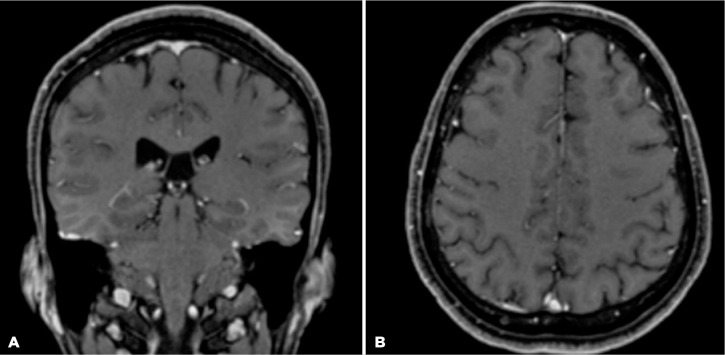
(A) Magnetic resonance images of the coronal and (B) axial frames after
